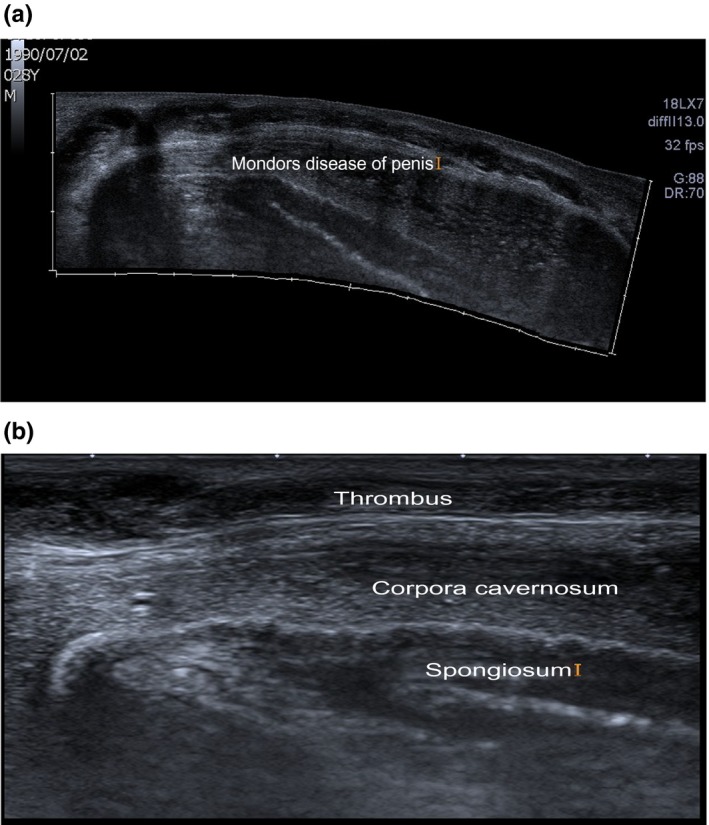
A, Panoramic ultrasound view showing the extensive thrombus from base of the penis to the corona. B, Sonographic image of the penis showing the thrombus, corpora cavernosum, and the spongiosum
